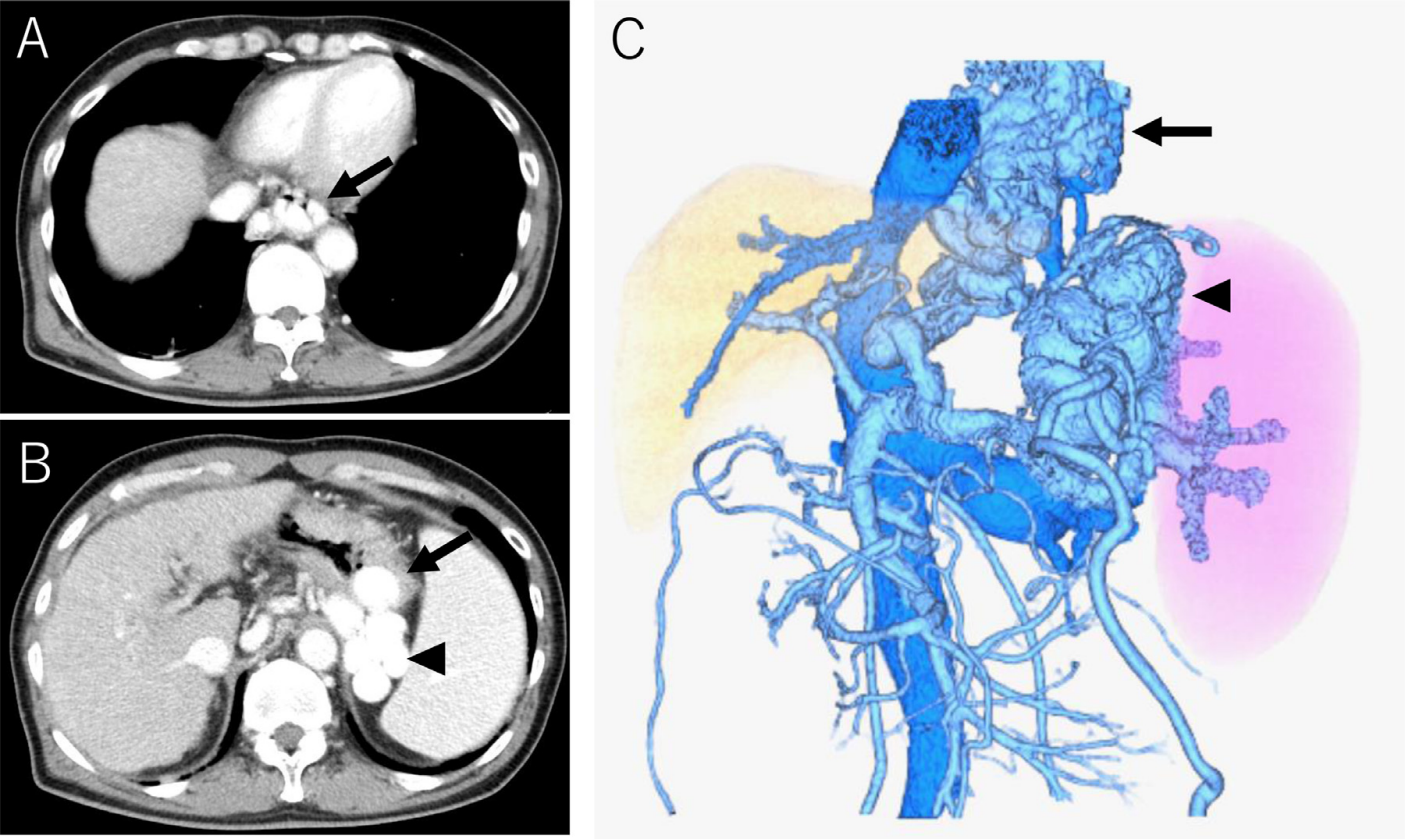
Contrast-enhanced CT and 3D-CT reconstruction image before treatment. (A) Contrast-enhanced CT before treatment shows giant esophageal varices (black arrow). (B) Contrast-enhanced CT before treatment shows gastric varices (arrow) and giant gastrorenal shunt (arrowhead). (C) 3D-CT reconstruction image before treatment shows giant esophageal varices (arrow) and gastrorenal shunt (arrowhead) with severe splenomegaly. The S/L ratio is 1.1.
